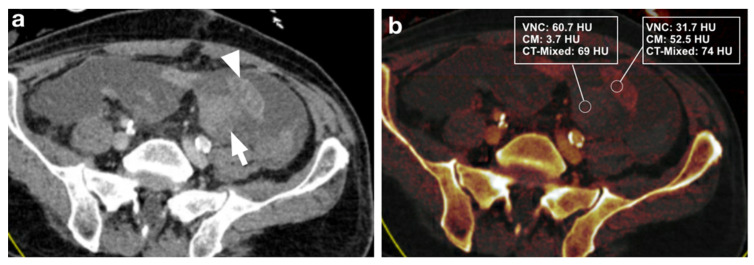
63-year-old male patient after a two-stage colectomy following mesenteric infarction with intestinal ischemia. (a) Intra-abdominal hematoma (arrow) adjacent to a small bowel loop (arrowhead). (b) Measurement of iodine maps shows a difference in VNC and iodine values (dual-energy CT) between hematoma and intestinal loops, but no difference in DE-LB.
